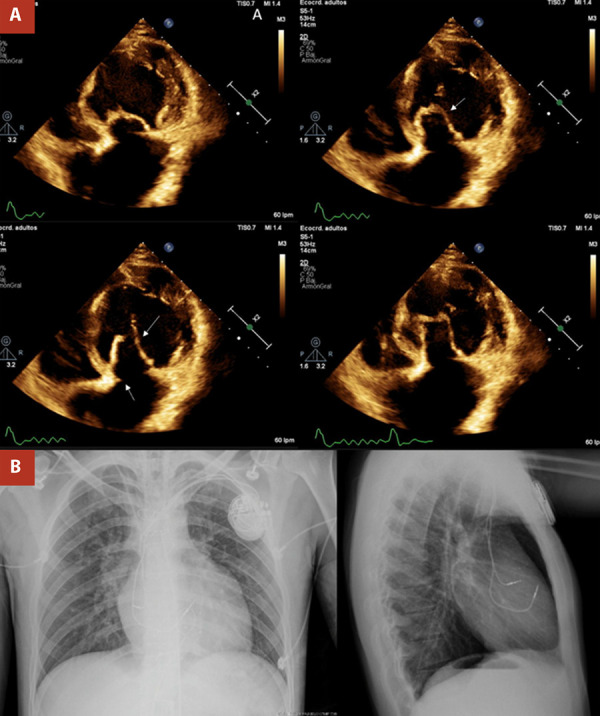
(A) Two-dimensional and Doppler echocardiogram. Arrows mark an Ebsteinoid systemic atrioventricular valve with severe insufficiency and a supravalvular stenosing ring. (B) Chest X-ray demonstrates pacemaker leads in the right heart chambers.
